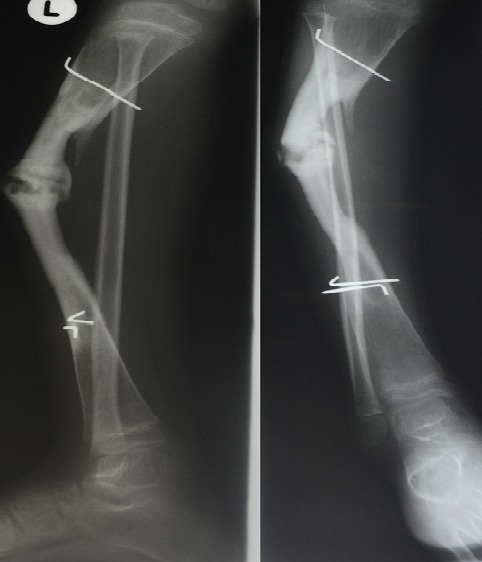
Lateral and AP radiograph of the right leg, showing a non-union fracture through a previous fibular graft in the tibia taken from the contralateral leg
